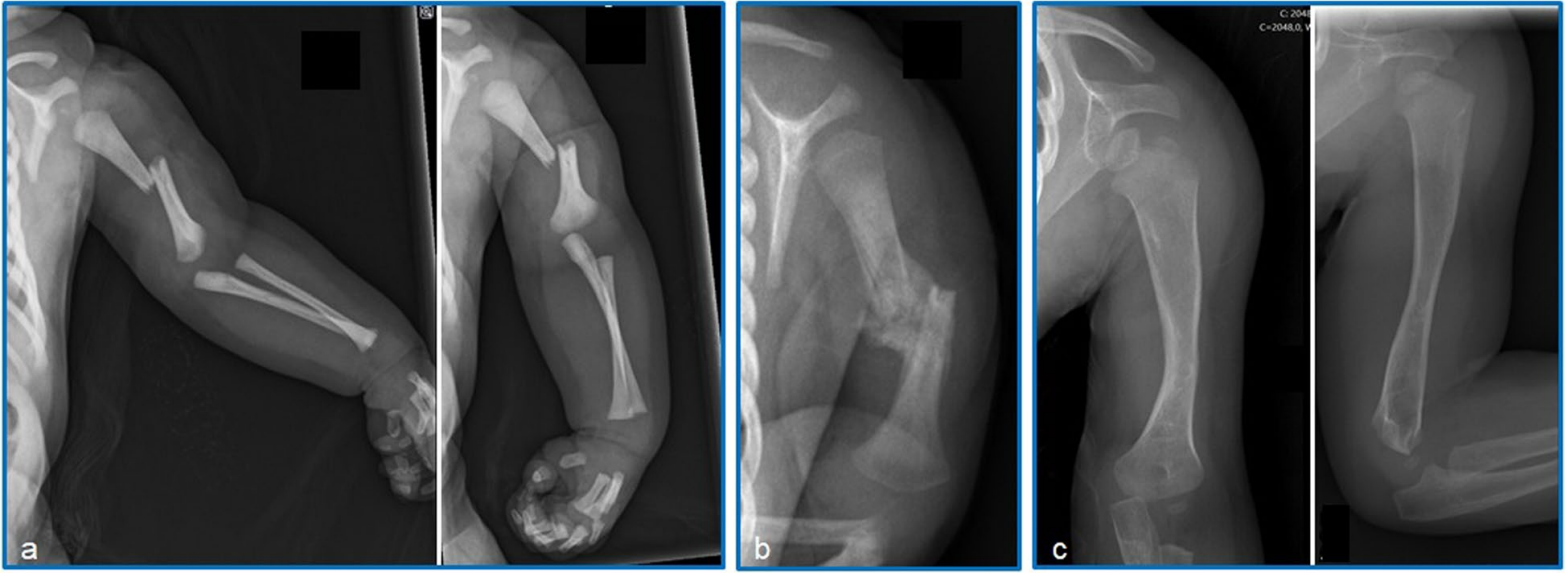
Male neonate, macrosomic (4024g), shoulder dystocia and instrumental delivery, with a right humeral shaft fracture, diagnosed on the 1st day of life, after limb deformity and humerus crepitus noticed immediately after delivery. Treatment was carried out with soft immobilization, placing the affected limb with the sleeve of a long-sleeved shirt attached to the chest with the elbow at 90º flexion. a X-ray on day 1 showing a right middle shaft humerus fracture with significant displacement. b At 3 weeks follow-up: X-ray with evidence of bone callus and indication for passive and active mobility of the right upper limb. c At 10 months follow-up: the patient was clinically without symptoms or restrictions and the X-ray shows clear evidence of consolidation and remodeling of the fracture
